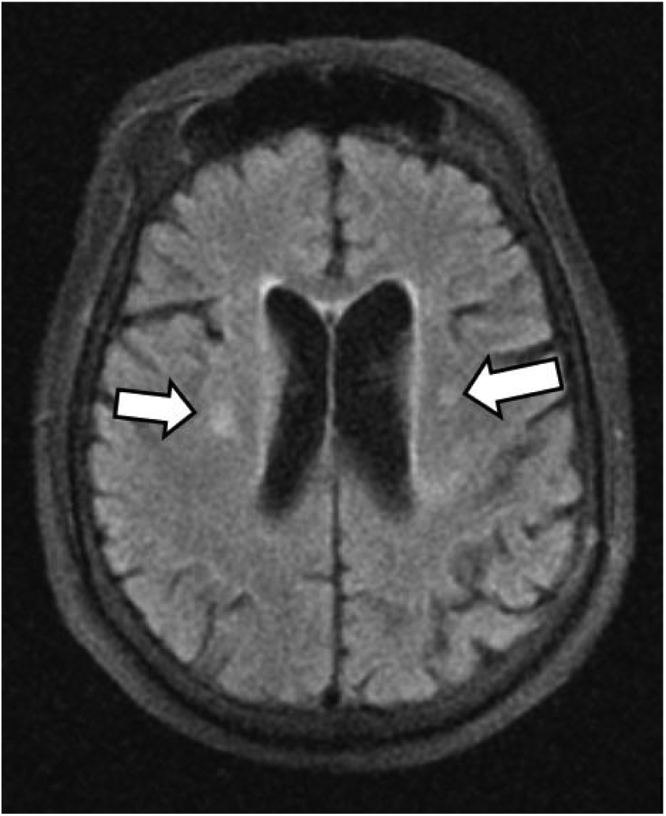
Follow-up non-enhanced MRI brain; Axial FLAIR sequence demonstrates stable size and distribution of white matter changes in the brain. (White arrows).
